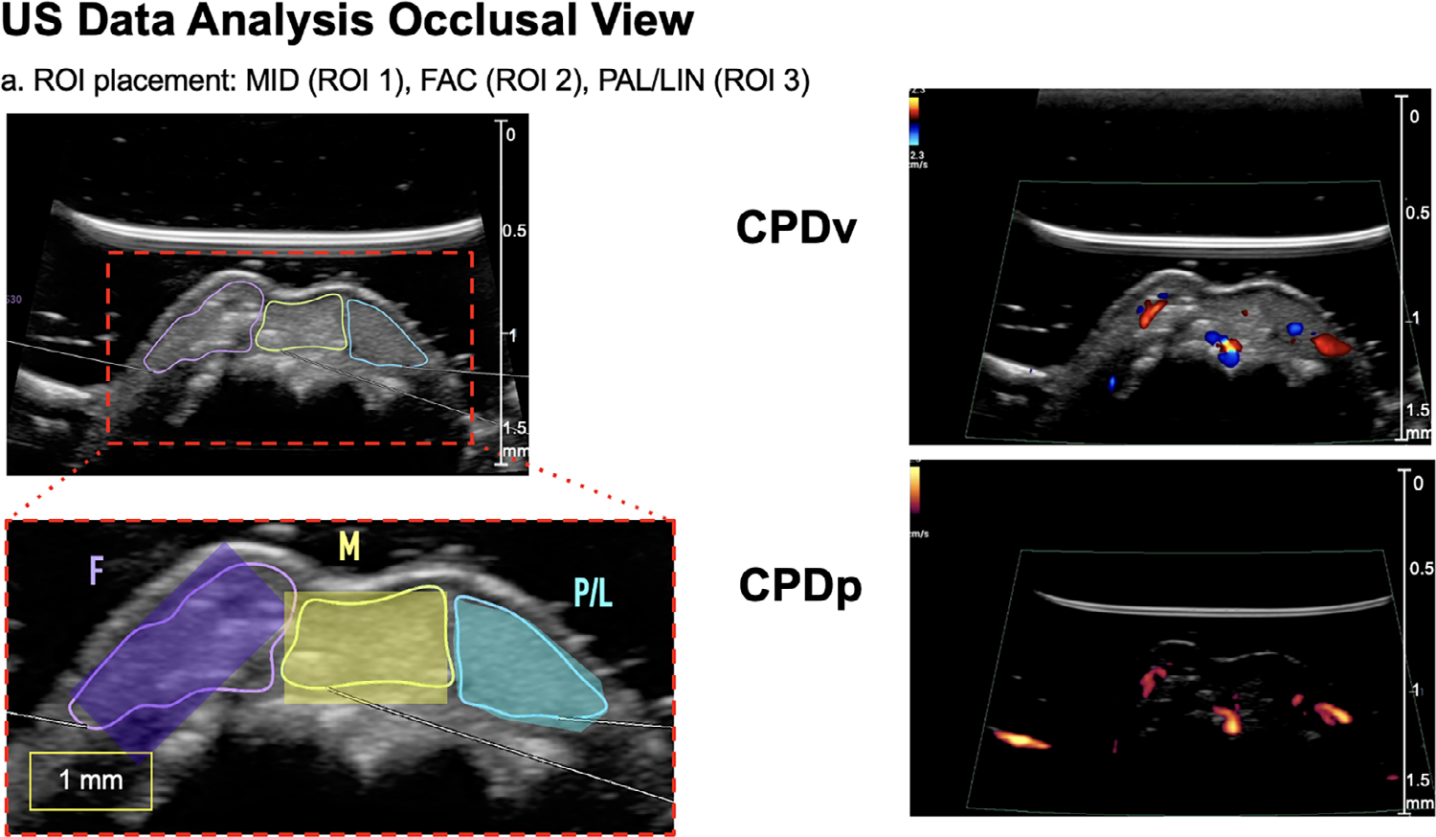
Edentulous ridge at 5 months after alveolar ridge augmentation was imaged with ultrasound (US) using a linear array (L30‐8, Mindray/Zonare) with 64‐µm axial resolution, second harmonic imaging (12‒24 MHz), and spatial compounding. B‐mode images (left) were captured. Color flow velocity (right top) and power images (right bottom) were obtained from occlusal view, with color flow recorded as a 90 dB B‐mode gain cine‐loop and power mode as a 40 dB B‐mode gain cine‐loop. Three regions of interest (ROIs) were defined as an example: mid‐occlusal (ROI 1), facial (ROI 2), and palatal/lingual (ROI 3), and color pixel density was calculated to indicate blood perfusion.
